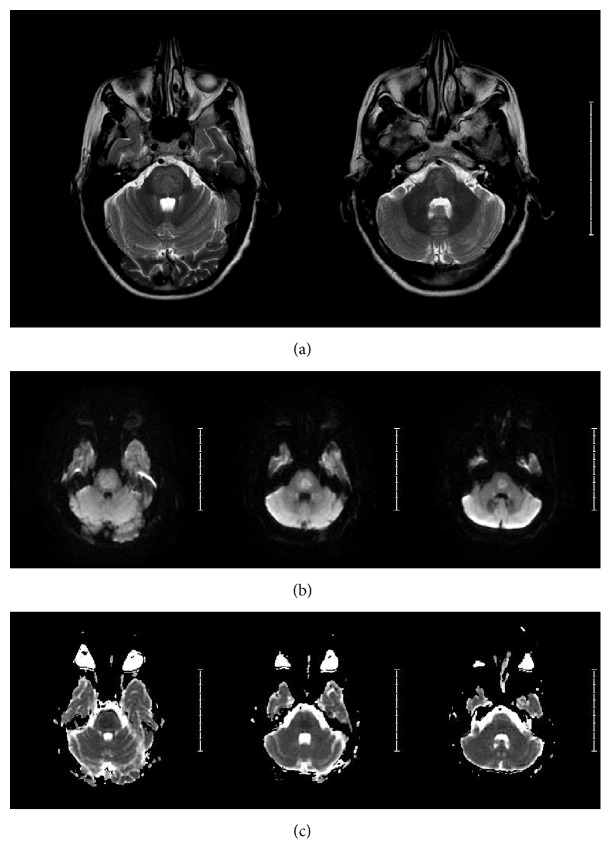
MRI with and without contrast on hospital day 15. T2-weighted MRI shows mild diffuse hyperintense signal changes in the pons (a); DWI sequence shows restricted diffusion (b) with corresponding ADC hypointensity (c) in central pons. These findings were not present when compared to MRI on hospital day 5 (Figure 3) and are consistent with central pontine myelinolysis.
